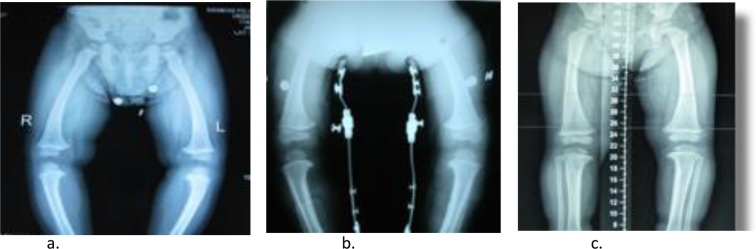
Anteroposterior radiographs of lower extremity, (a). Before treatment, (b). Before treatment with KAFO, (c) After treatment.
